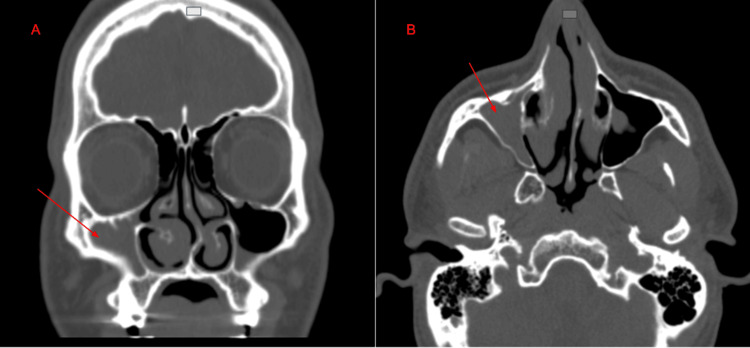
Non-contrast CT imaging of the paranasal sinuses revealing right maxillary sinus opacification and associated findings. Coronal (A) and axial (B) views of a non-contrast CT scan of the paranasal sinuses showing complete opacification of the right maxillary sinus (red arrows). Additional findings include atelectasis of the right uncinate process and inward bowing of the medial maxillary sinus wall.
